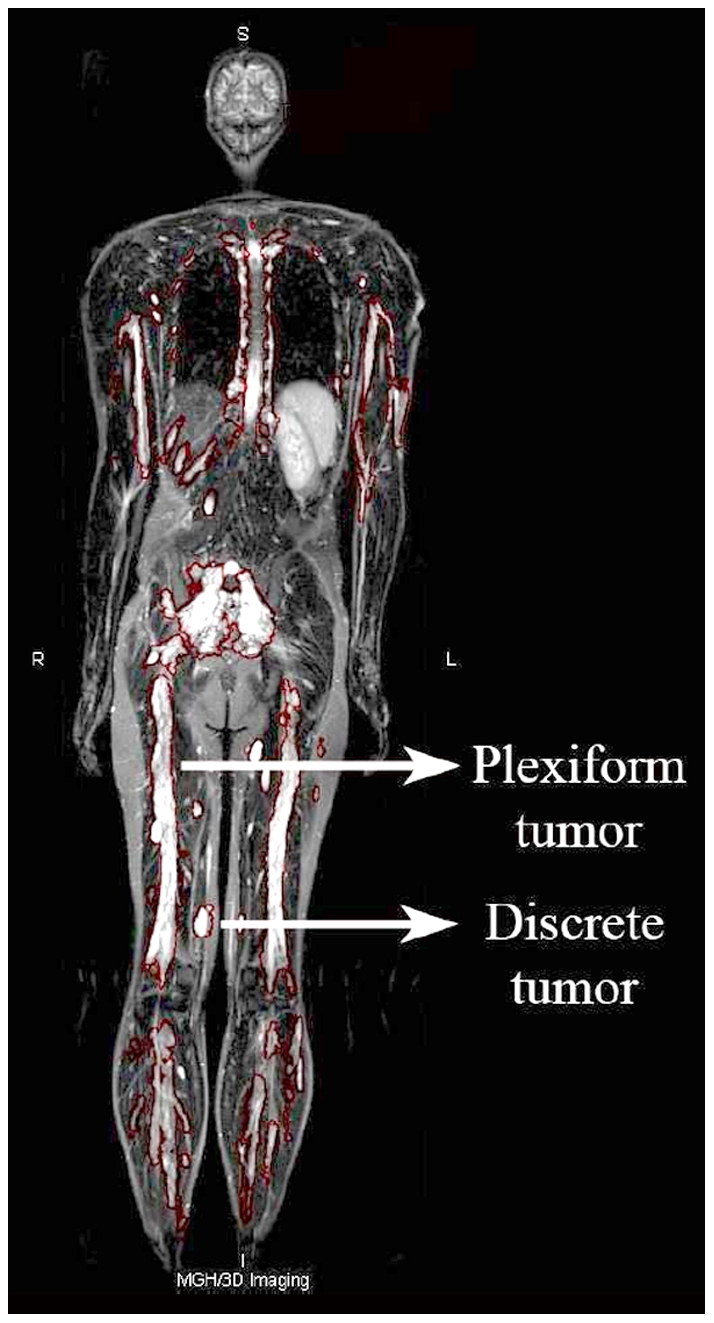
Appearance of internal nerve sheath tumors on Whole-Body MRI. Tumor type was defined according to the radiologic appearance without need for pathological diagnosis. Tumors that were locally circumscribed on MRI scan were classified as circumscribed tumors and those that were invasive or involved multiple nerves were classified as plexiform tumors.
